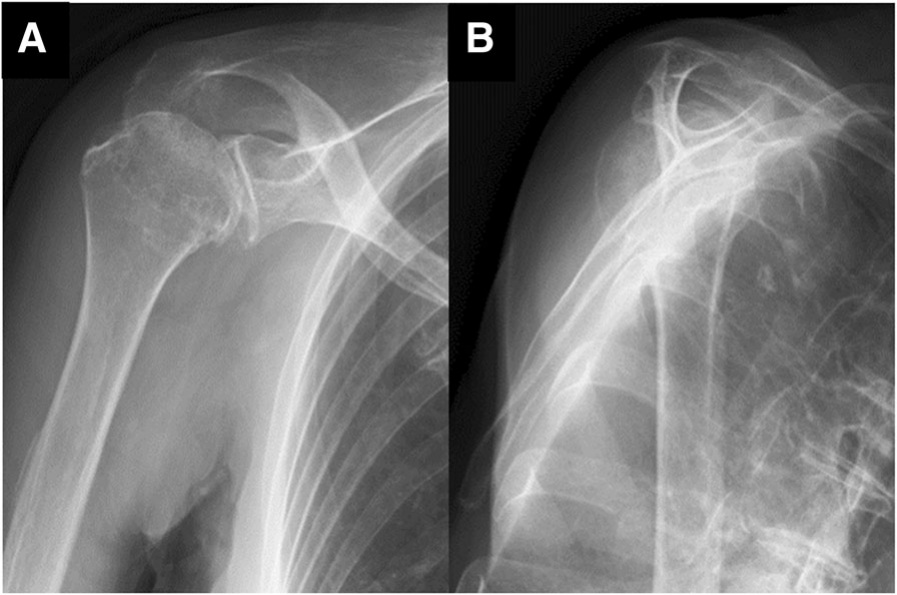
X-ray radiography images after reduction revealing that the dislocation was reduced (a, b)
